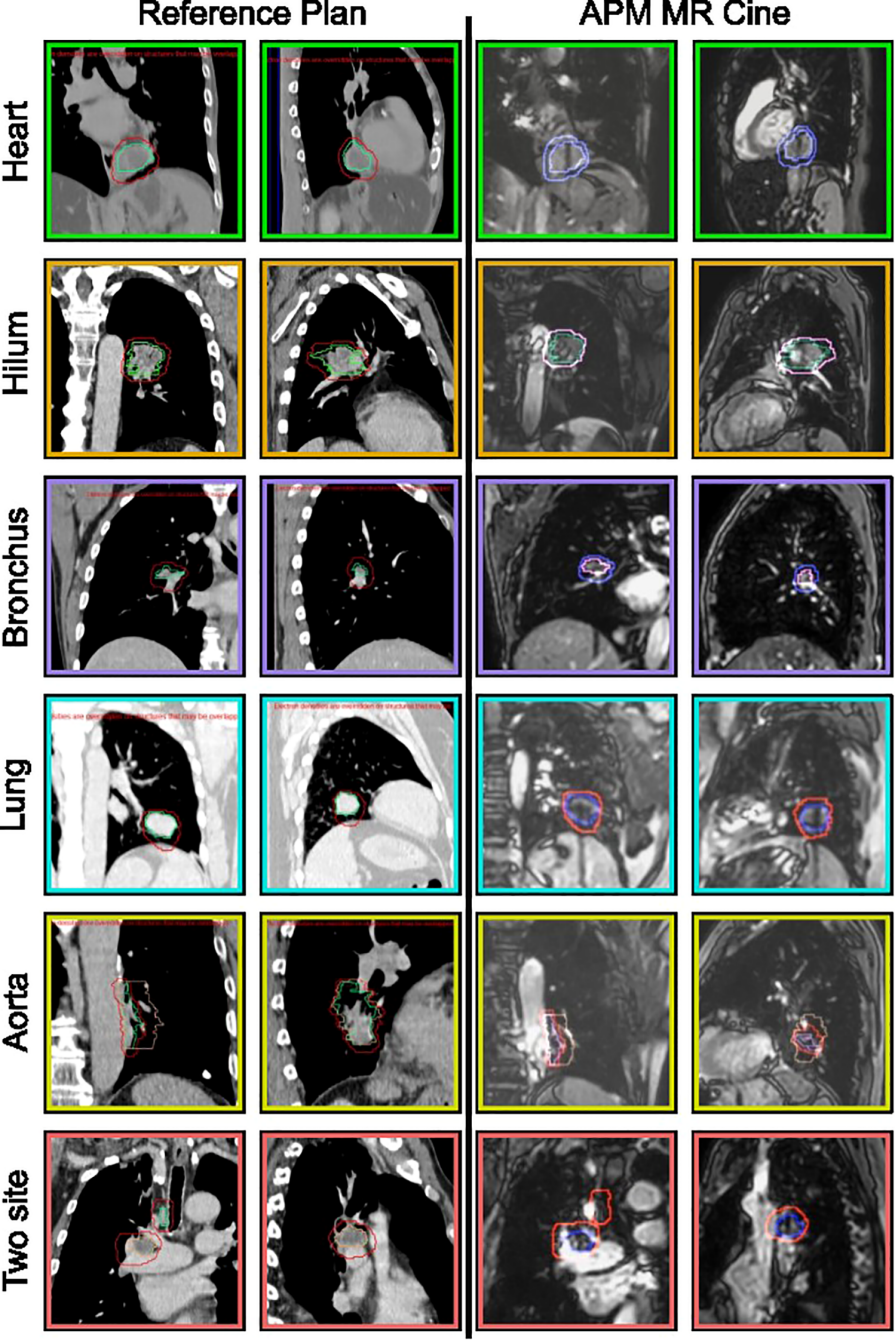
Subset of lung sites treated using MR cine gating on the Elekta Unity MRLinac. The reference CT is shown in columns 1 and 2 with the coronal and sagittal views of the target, green, gating envelope, red, and surrogate (peach, if applicable). Columns 3 and 4 are the MR cine analogs of the reference plan showing the tracking structure, surrogate (if used), and gating envelope.
